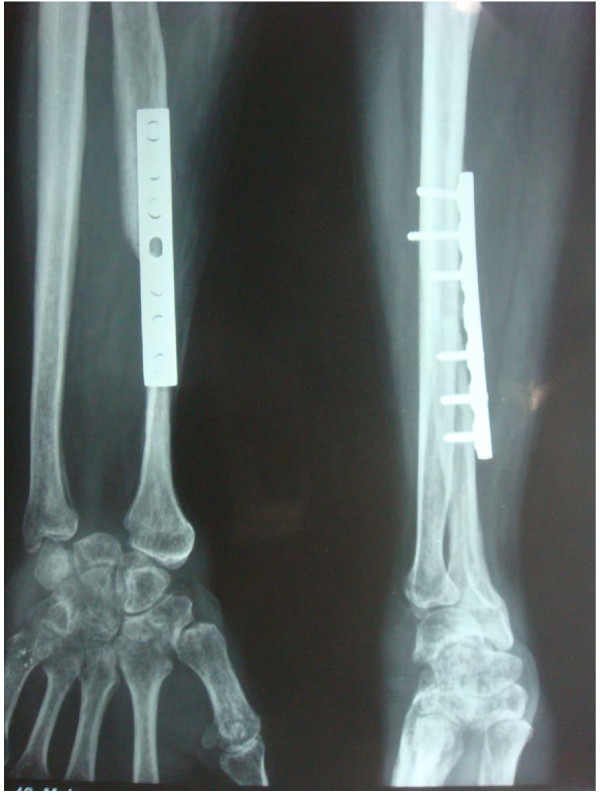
Follow up radiographs showing union of the fibular graft with the radius.
